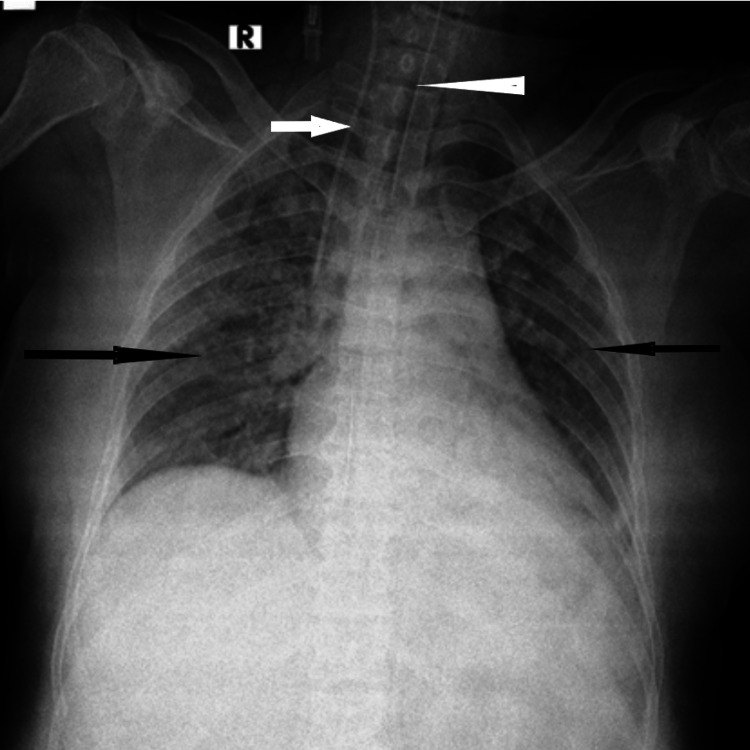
Case 1 Thirty-five-year-old COVID-19-positive patient had a history of dyspnoea for 10 days. The patient underwent invasive ventilation. Diffuse patchy air space opacities indicated by black arrows are noted involving bilateral lung parenchyma on portal chest X-ray suggestive of pneumonia. The central line indicated by a white arrow and the endotracheal tube indicated by an arrowhead can be seen.
